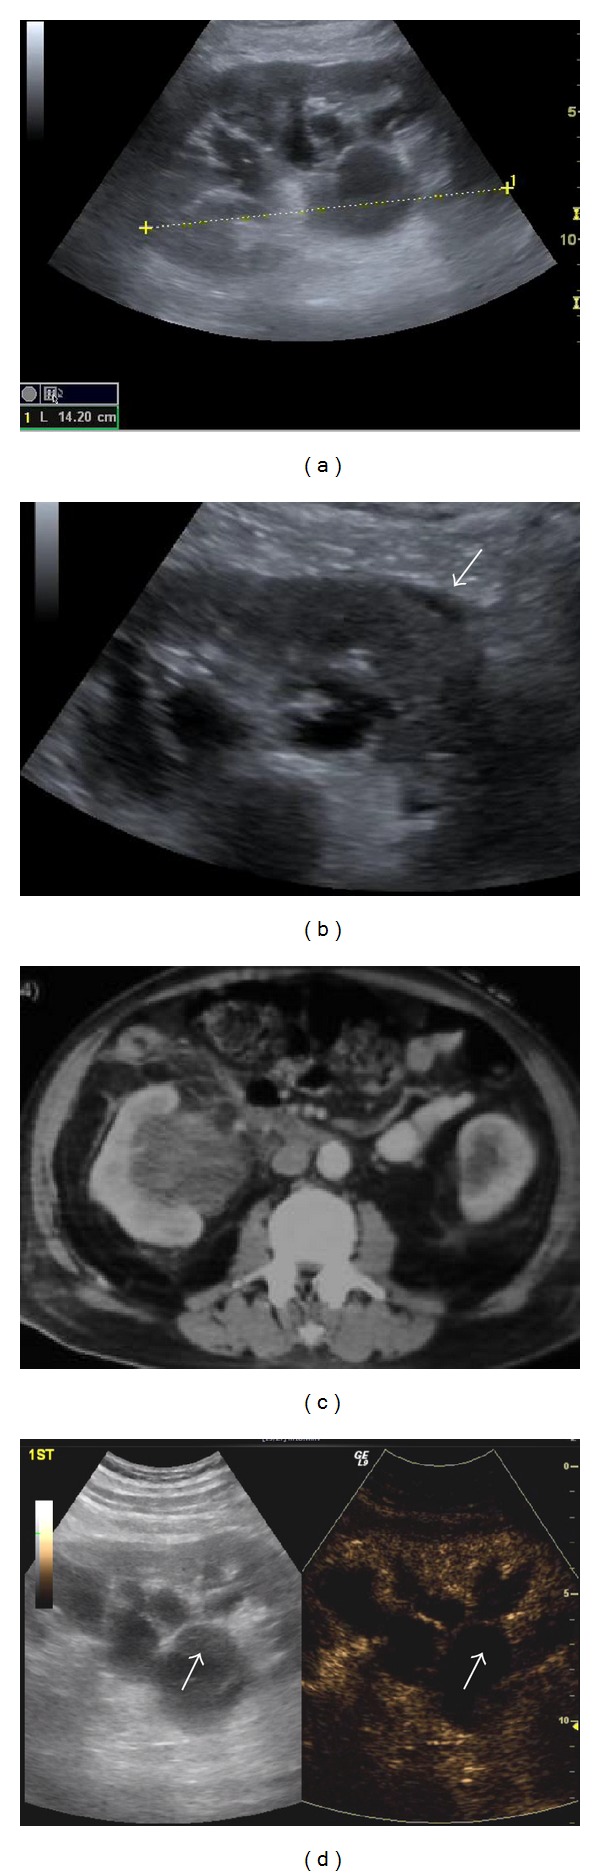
Pyelonephritis: B-mode US images an enlarged right kidney (a) with a dilated pelvicalyceal system, containing echogenic material. A small perinephric collection is also seen (arrow in (b)). CT (c) confirms these findings. On CEUS (d) the echogenic material in the collecting system (arrows) does not enhance, suggestive of a purulent, non neoplastic nature. Note the difference from transitional cell carcinoma enhancement (Figure 3).
